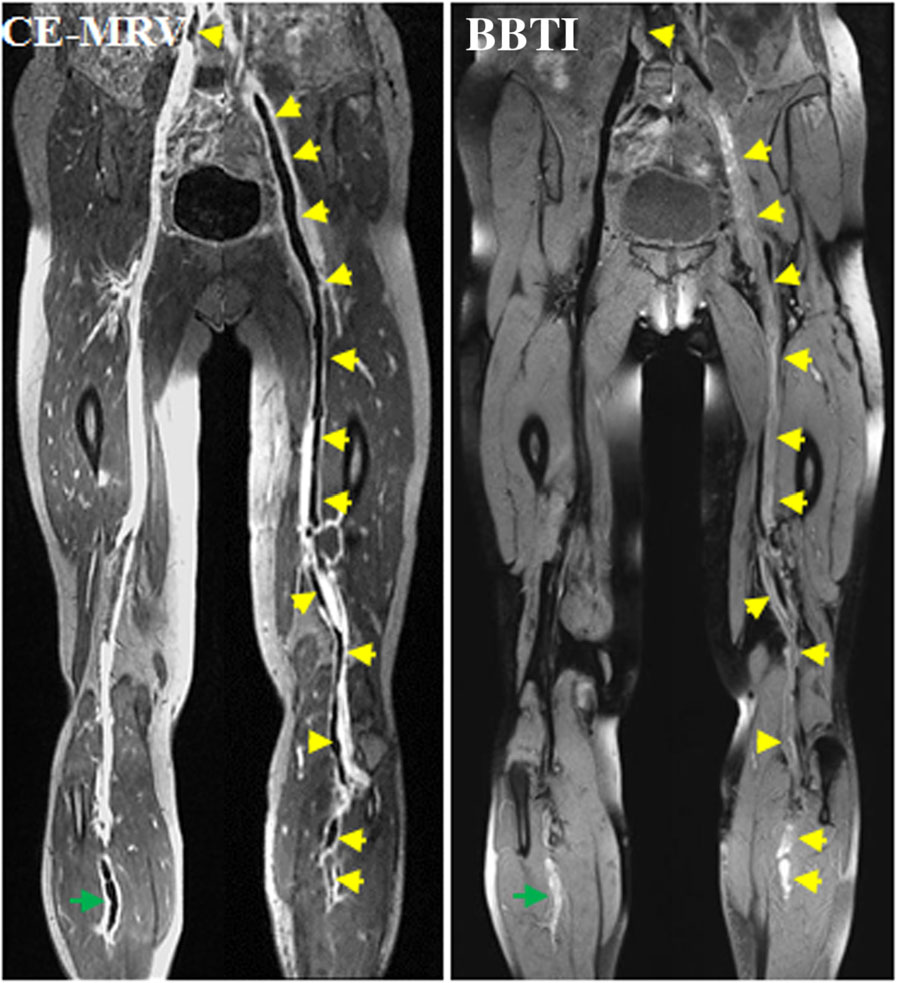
Example images obtained in 50-year-old woman with DVT symptom onset at 3 days. The thrombus (green arrows) was detected by BBTI at the asymptomatic right leg, which was missed by the initial ultrasound and confirmed by CE-CMRV. Most parts of the thrombi (yellow arrows) appeared as isointense signals on the BBTI images. The locations and sizes of the thrombi between BBTI and CE-CMRV matched
